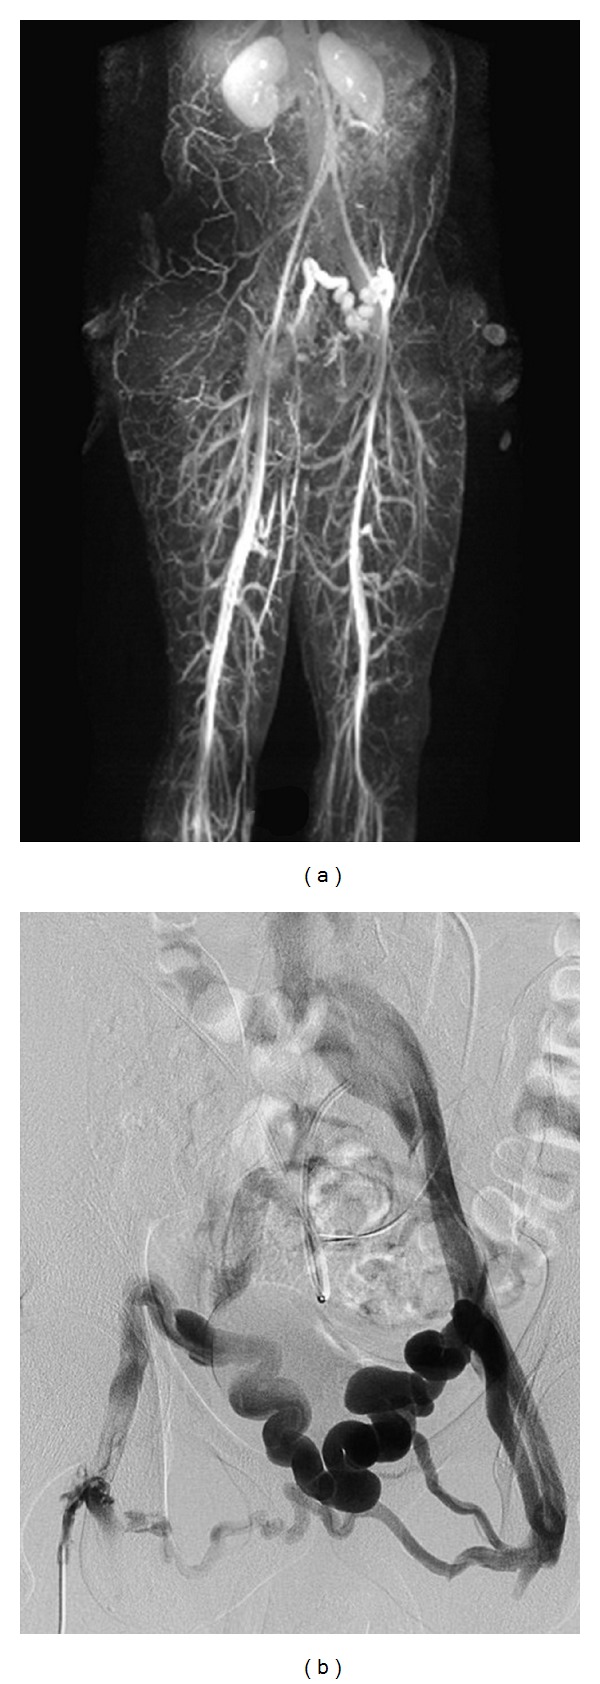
MRI with contrast demonstrating abnormal iliac fossa varicosities (a) Conventional right femoral venogram demonstrating large right to left cross pelvic collaterals to the left femoral system (b).
